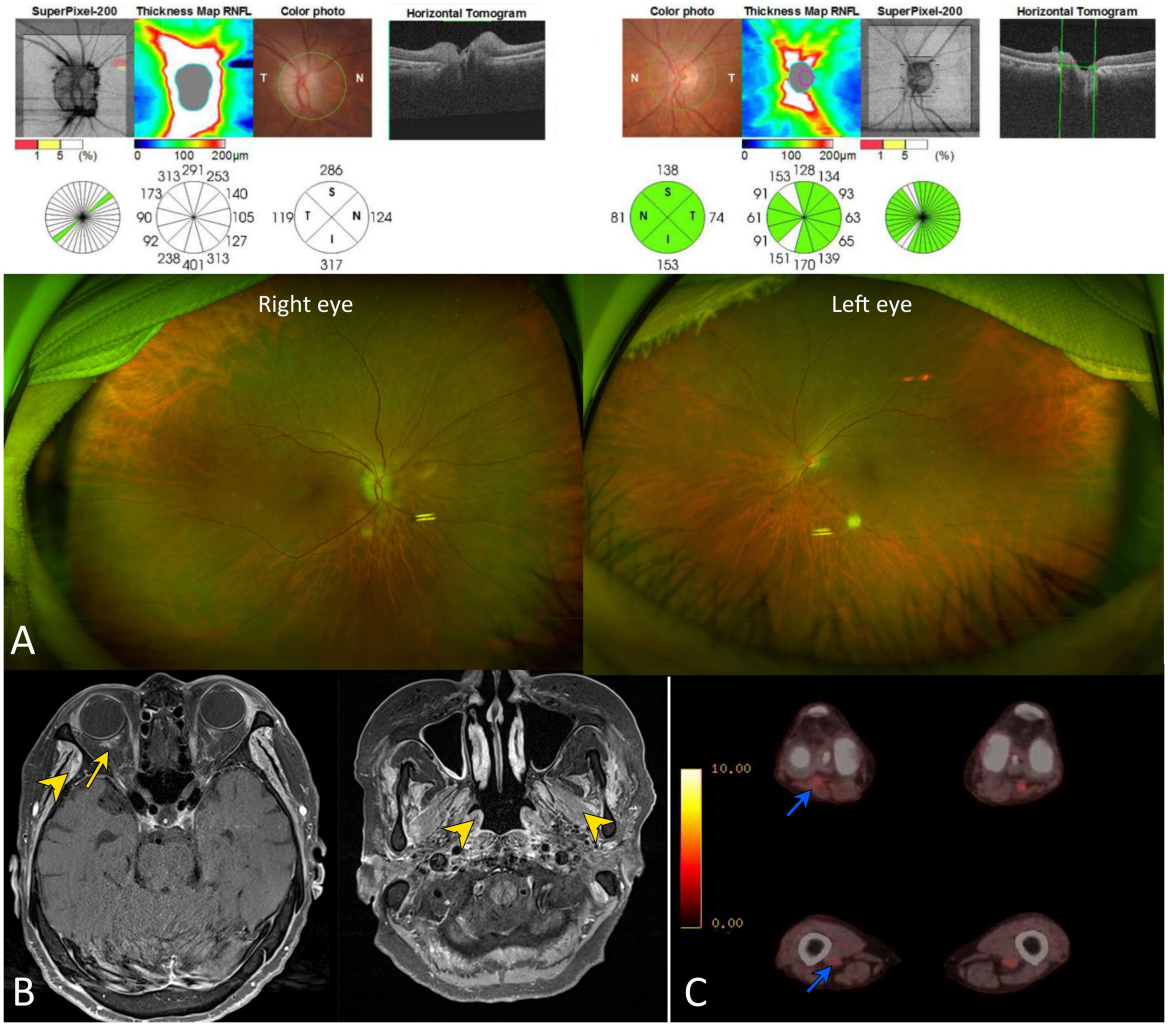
Neuro-ophthalmologic and imaging findings of our patient. (A) Scanning laser ophthalmoscopy and optical coherence tomography show diffuse disc swelling in the right eye. (B) A gadolinium enhancement was documented around the right optic nerve sheath on gadolinium-enhanced T1-weighted images (yellow arrow). This finding suggests that the posterior ciliary arteries may be affected, secondary to adjacent inflammation. Multifocal enhancements were also found in bilateral temporalis, masseter, and pterygoid muscles (yellow arrowheads). (C) Whole-body positron emission tomography (PET) showed an abnormal glucose uptake in the popliteal and femoral arteries (blue arrowheads).
